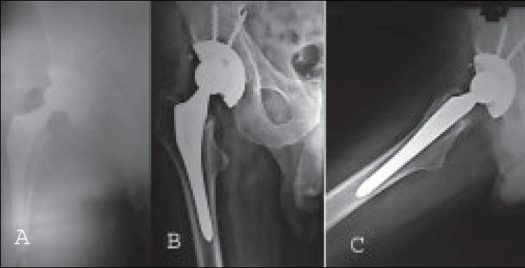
Immediate postoperative Xrays (a) of a 42-year-old male with uncemented THR with well fixed prosthesis. X-rays AP and lateral views (b and c) at five-year follow-up show no osteolysis
